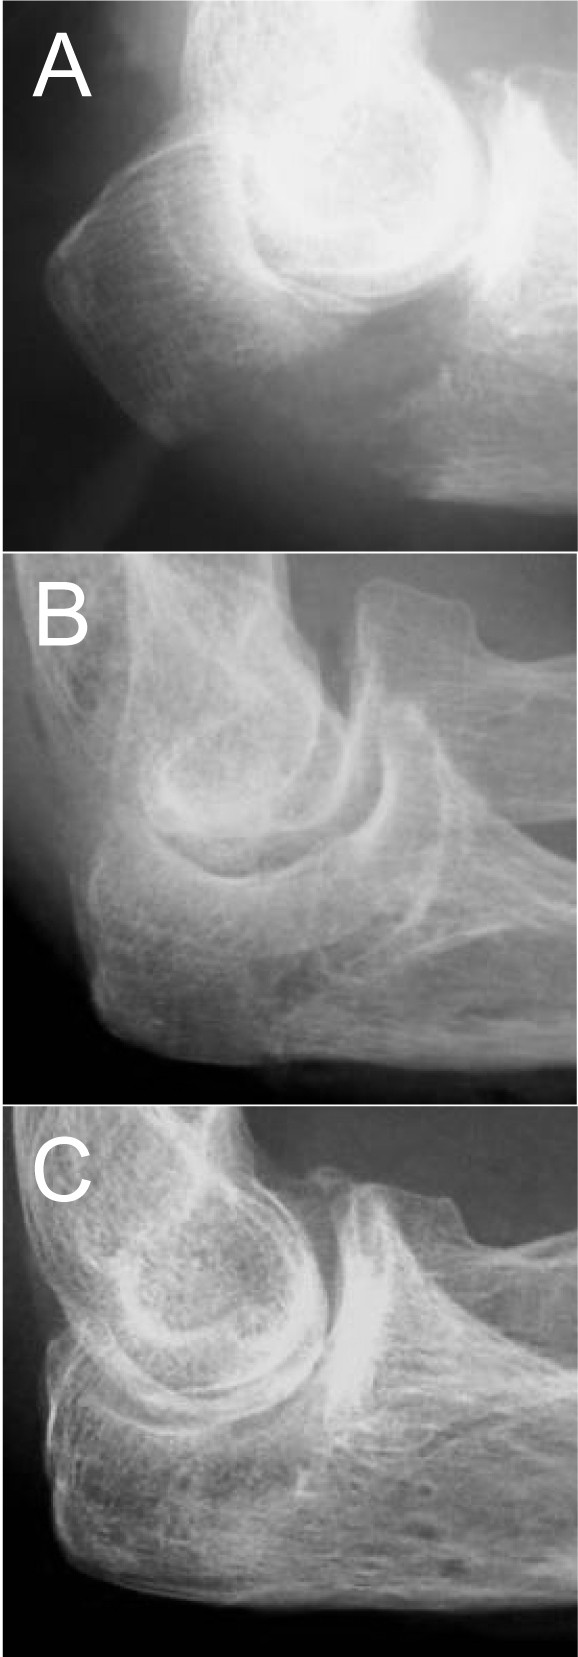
Radiographs of case 2. (A) Lateral radiograph of preoperative period. (B) Lateral radiograph of intraoperative period. (C) Radiograph of 1 year postoperative period.
